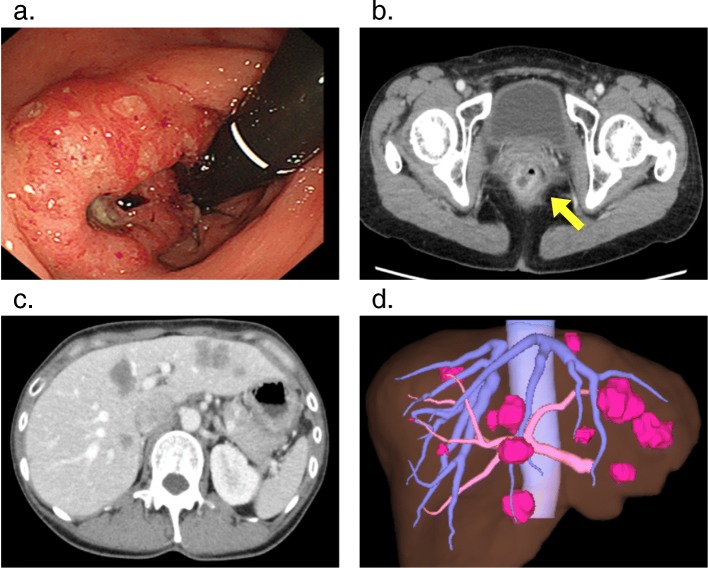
CT imaging and a colonoscopy before neoadjuvant chemotherapy. a Primary rectal cancer on a colonoscopy. Type 2 rectal carcinoma. No bleeding or occlusion is observed. b Axial view of primary rectal cancer (arrow) on CT. Contrast-enhanced wall-thickening (20 mm) is seen in the lower rectum. There were no signs of occlusion. c Axial view of multiple bilobular liver metastases on CT. d Multiple liver metastases on 3-dimentional image from CT. Lesions are bilobular. CT, computed tomography
